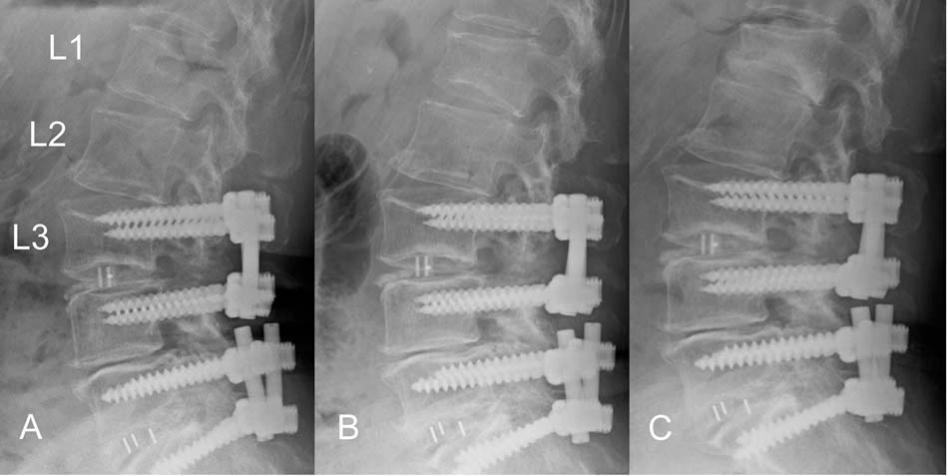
Time course of a vertebral fracture at L1. (A) Radiographic image obtained just before the vertebral fracture occurred. (B) The vertebral fracture had occurred at L1 4 months before surgery. (C) Radiographic image obtained when the patient presented with symptoms of radiculopathy shows no apparent posterior slip of the L2 vertebra. The L1 vertebral body was deformed into a wedge shape.
